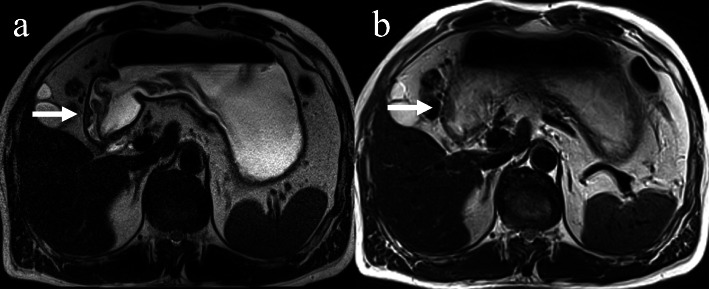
Comparison of two T2-weighted MR images of a patient with ulcerative moderately to poorly differentiated adenocarcinoma at greater curvature of the gastric antrum showing superiority of HASTE (a) versus BLADE (b). Localized thickening of gastric antrum wall can be seen (arrow). While some artifacts are present in both images, image artifacts were rated as “not hampering image evaluation” (score 2) for T2- HASTE, but rated as“hampering image evaluation” (score 1) for T2-BLADE. Lesion conspicuity was rated as “excellently delimitable” (score 4), and“less than 25% of lesion borders definable” (score 1), respectively. In addition, T2- HASTE image showed an ulcer with crater shape, and the submucosa high signal line, which is of great value for diagnosis and staging, but not on T2-BLADE image
